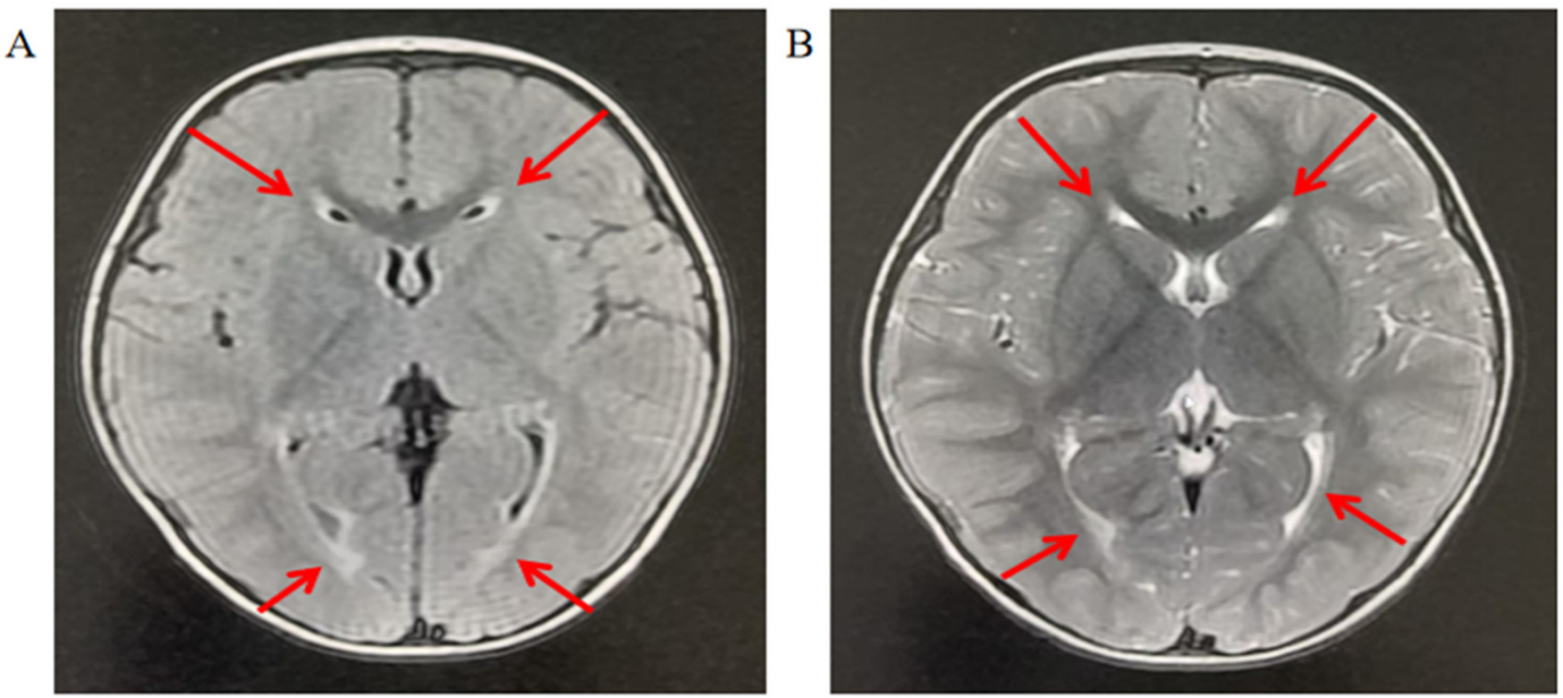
Brain MRI of the patient indicates myelination dysplasia. (A) T2-weighted imaging: The arrowhead denotes a region displaying abnormally persistent hyperintensity, suggesting delayed or disrupted myelination. This finding is consistent with hypomyelination, characterized by reduced myelin content and disorganized axonal maturation. (B) T1-weighted imaging: The arrow indicates a region with persistently hyperintense signal, further corroborating hypomyelination as immature white matter retains high T1 signal intensity until full myelination is achieved. The T1–T2 signal discrepancy (“T1 bright, T2 bright” paradox) is pathognomonic for developmental white matter abnormalities.
